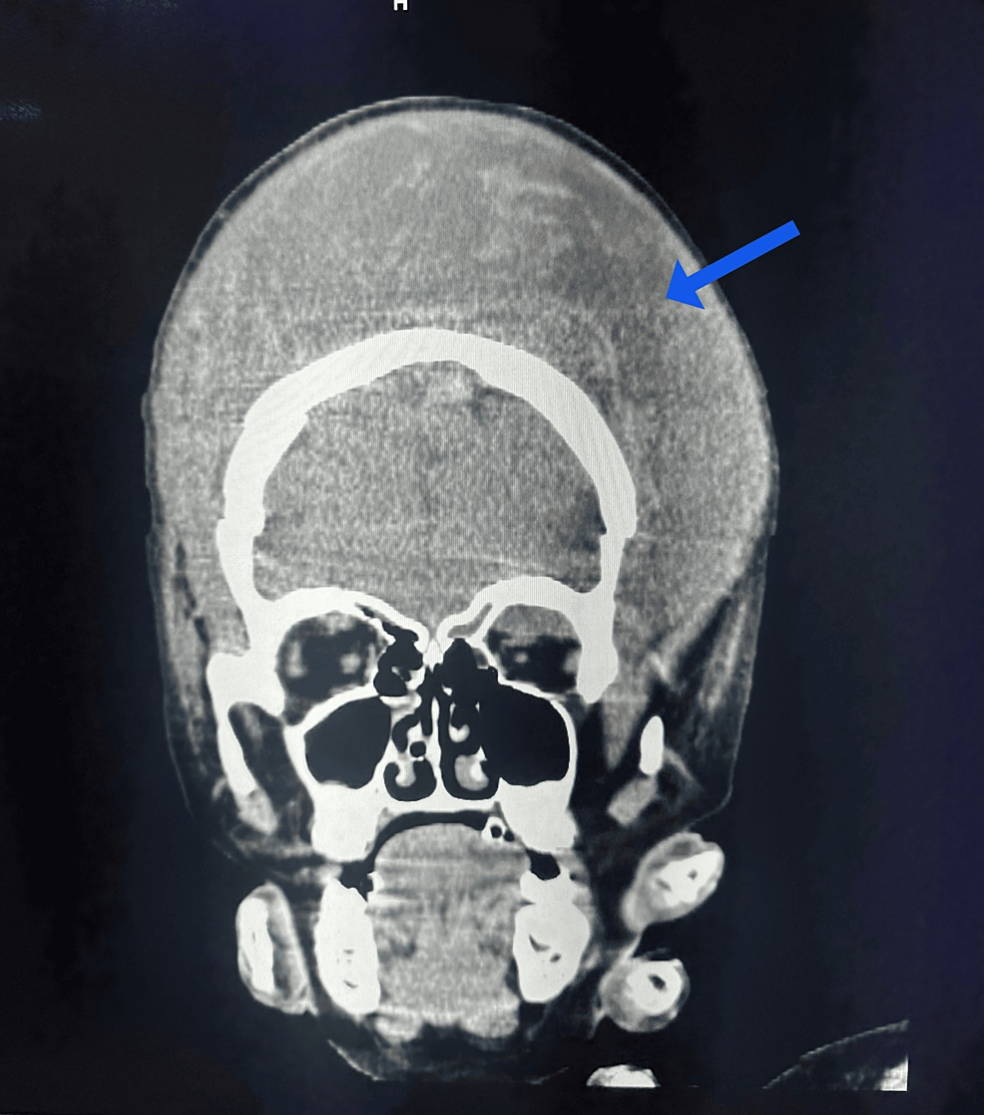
Non-contrast computed tomography coronal section showing the large subgaleal hematoma in the bilateral frontoparietal region, as shown by the blue arrow
